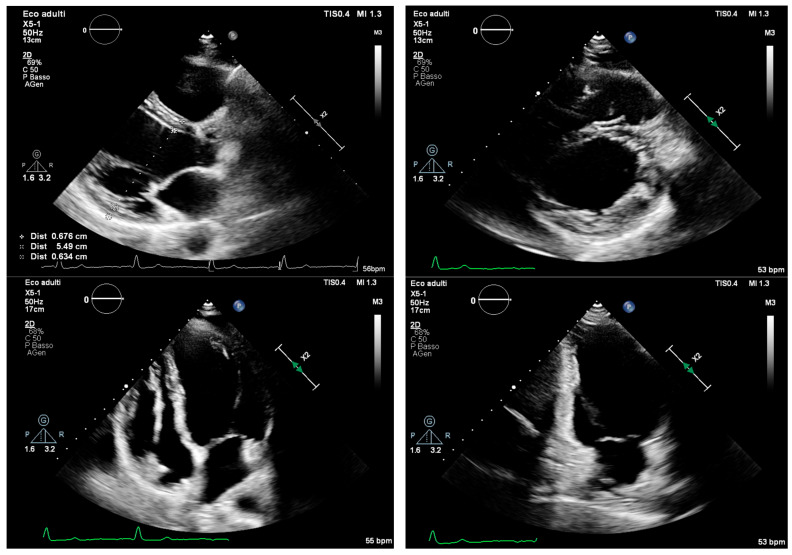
Echocardiographic images of a patient at 38 weeks of gestation affected by dilated cardiomyopathy with left ventricular systolic function at the lower limit of normal. She had a history of syncope and previously documented second-degree atrioventricular block (type 2:1), which alternated with trifascicular block. The patient also experienced episodes of non-sustained ventricular tachycardia and paroxysmal atrial fibrillation. Genetic testing revealed a variant of uncertain significance in the myopalladin gene. Pregnancy did not result in a deterioration of left ventricular systolic function or an increase in ventricular volumes.
